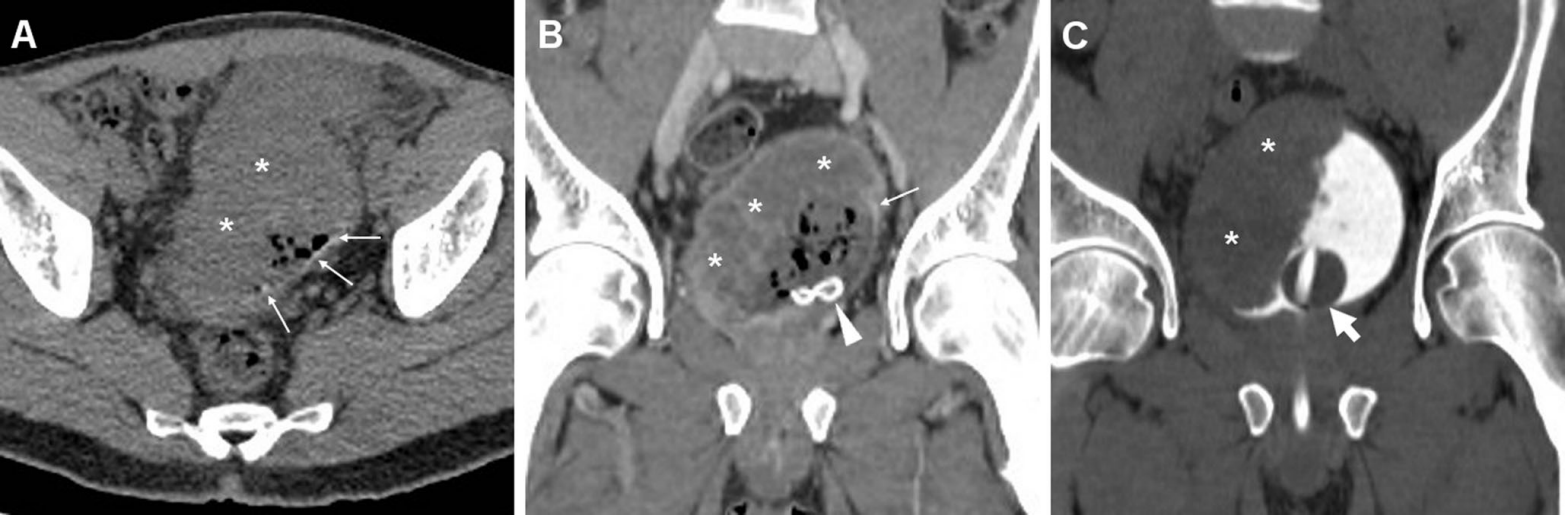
Schistosomiasis with superimposed squamocellular carcinoma in a 48 year-old Gambian man with pelvic pain and tenderness, dysuria and difficult urination. Unenhanced (a) and post-contrast (b) CT images showed marked asymmetric solid mural thickening (*), with poor enhancement along the anterior right lateral and superior bladder aspects. Additional findings included thin calcifications (*thin arrows*) along the left posterolateral bladder wall, and intraluminal stones (*arrowhead*). CT-cystography (c) with retrograde contrast filling and cystoscopy confirmed extensive bladder occupation by tumour (*) [Partially reproduced with permission from Ref.[37]]
